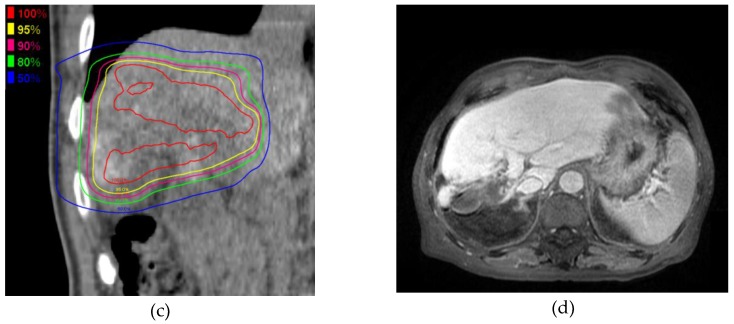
A 6.3 cm hepatocellular carcinoma (HCC) was treated by proton beam therapy (PBT) in a patient with Child–Pugh class A liver disease and right portal vein tumor thrombosis. (a) Early enhancement of the HCC was shown in the arterial phase of gadolinium ethoxybenzyl diethylenetriamine pentaacetic acid-enhanced dynamic magnetic resonance imaging (Gd-EOB-MRI); (b) The isodose lines were shown on planning CT (axial view); (c) This tumor was close to the colon (coronal view); (d) HCC was shown in the arterial phase of EOB-MRI 2 years after PBT.
